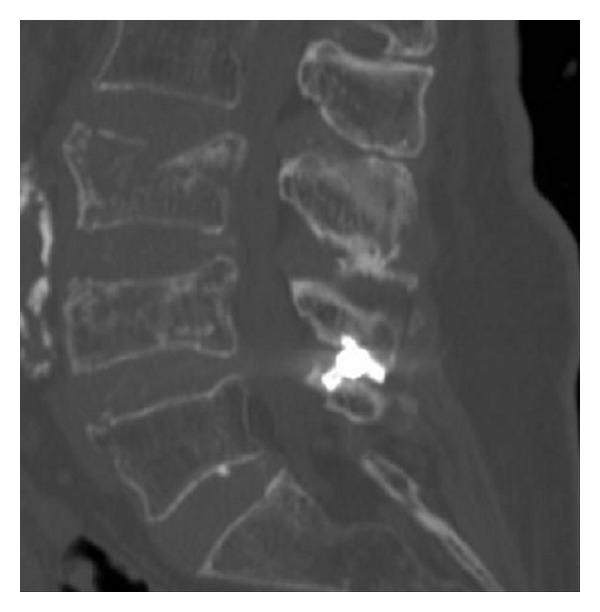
Sagittal CT image of the Aperius inserted at L4-L5 level.
